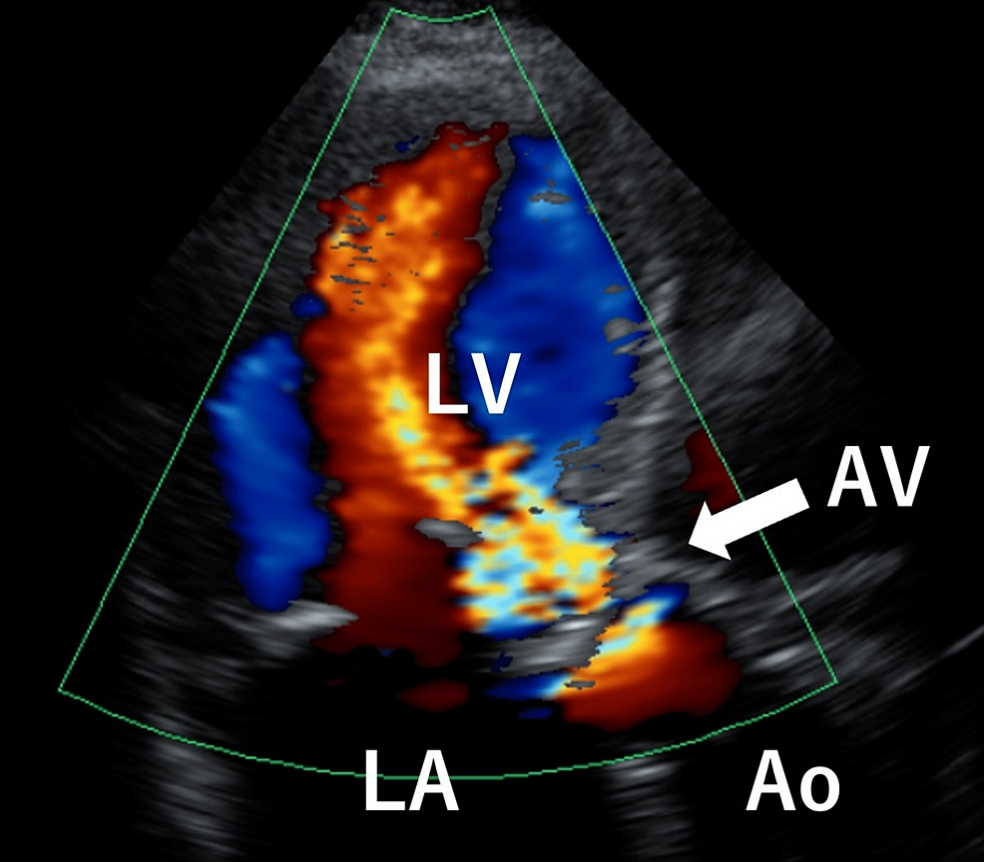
Echocardiography on admission showing severe aortic regurgitation (arrow). Ao, aorta; AV, aortic valve; LA, left atrium; LV, left ventricle.
